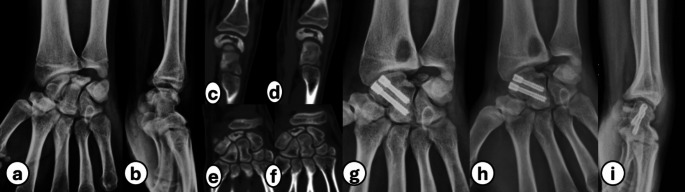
(a, b): AP and lateral wrist radiographs demonstrate stage IIIB KD with lunate collapse and fracture. (c-f): Sagittal and coronal CT slices show collapsed lunate with fragmentation. (g, h, i): AP, scaphoid, and lateral wrist radiographs after 7 months of follow-up reveal lunarectomy and complete healing of the scaphocapitate fusion site with evidence of bone graft harvesting site at the distal radius
